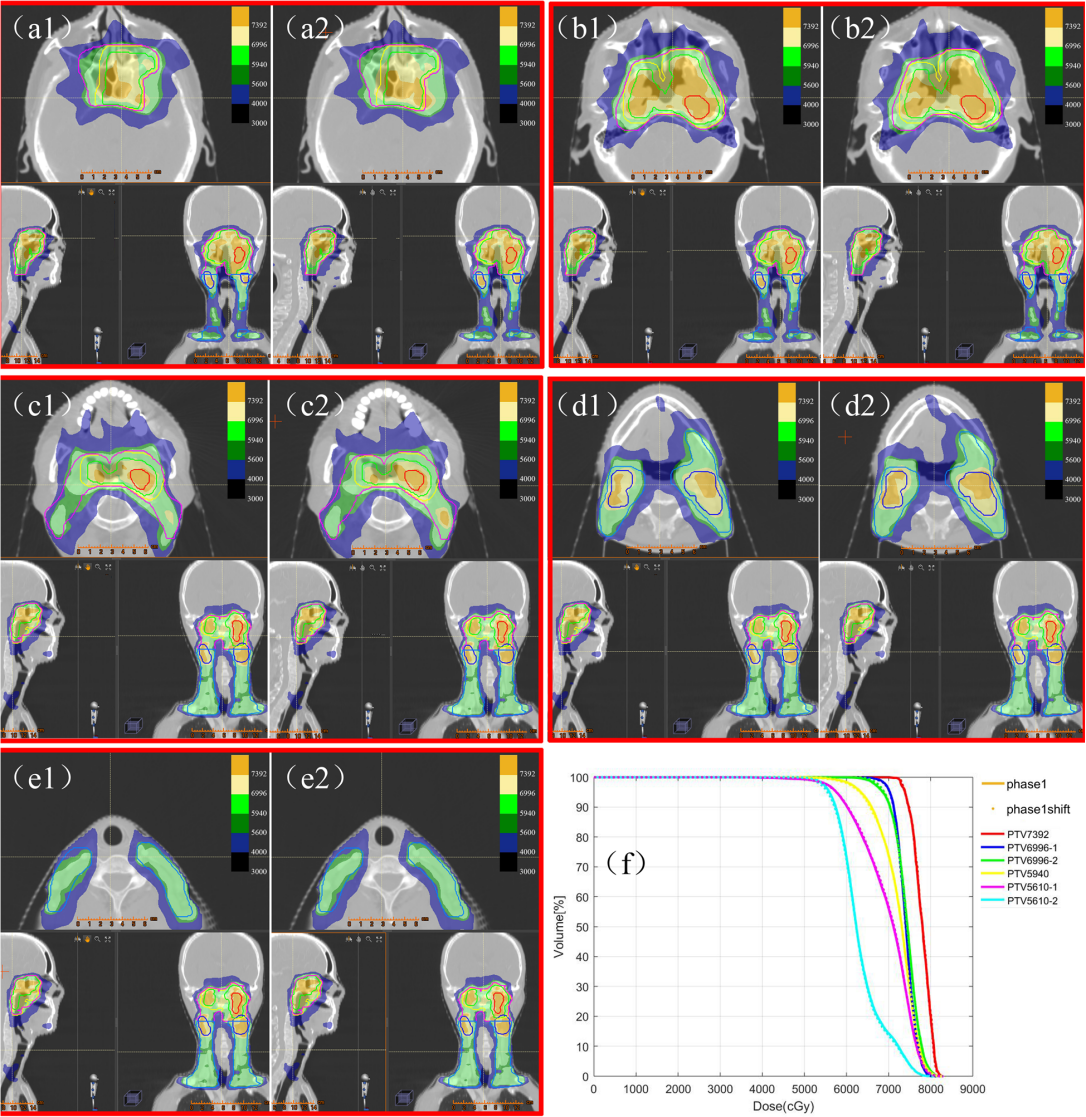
Dose comparison of the original plan and the shifted plan of case 2. (a1), (b1), (c1), (d1) and (e1) are multiple slices of dose distribution of the original plan. (a2), (b2), (c2), (d2) and (e2) are the dose distribution at the same location of the shifted plan according to the registration difference between F-CBCT and D-CBCT. (f) is the dose–volume histogram of the target volume from the original plan and the shifted plan
